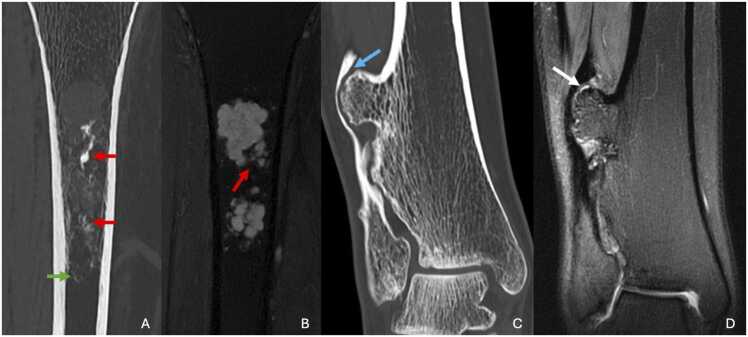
A,B. Enchondroma of the proximal third of the right tibia in a 50-year-old woman. C,D. Osteochondroma of the distal metaphysis of the right tibia in a 20-year-old woman (different patient). A. CT image in the coronal plane showing an osteomedullar osteolytic lesion with central popcorn calcifications (red arrow) and thin arc calcifications (green arrow), without endosteal scalloping. B. T2-weighted fat-suppressed MR image in the coronal plane showing a multilocuted lesion with cartilaginous matrix: hypersignal with some marked hyposignal corresponding to calcifications (red arrow). C. CT image in the coronal plane showing a pedunculated bony outgrowth presenting continuity with supporting bone. Notice the mass effect on the fibula (blue arrow) D. T2-weighted fat-suppressed MR image in the coronal plane showing a thin cartilaginous cap (white arrow).
